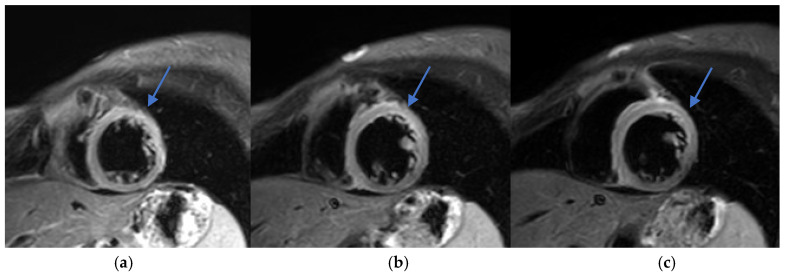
(a–c) T2-weighted short tau inversion recovery (T2-STIR) images. Increased signal intensity is noted in the anterior wall (arrows). The myocardium-to-skeletal muscle signal intensity ratio is approximately 3:1, consistent with myocardial edema.
